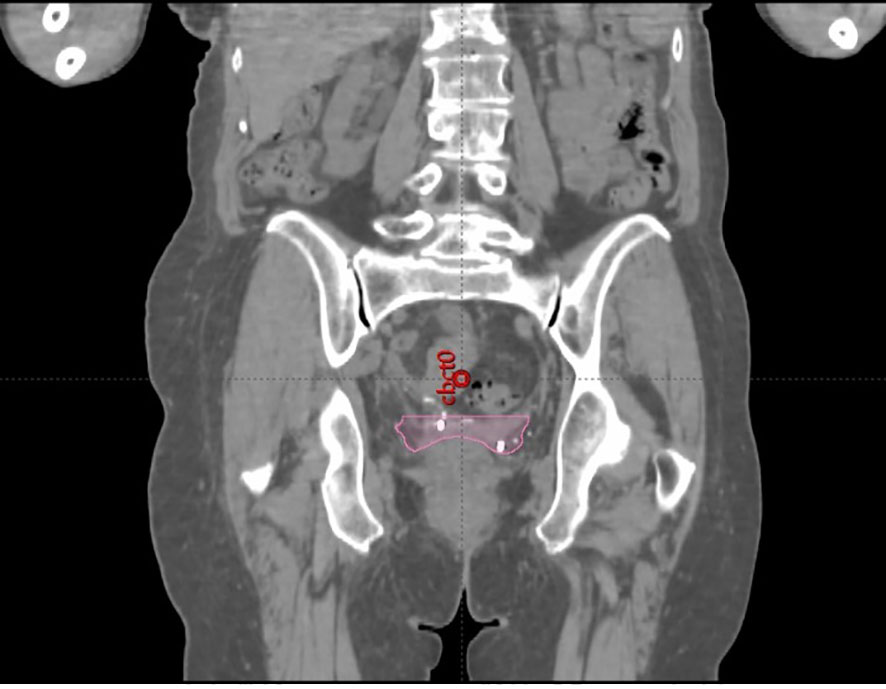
Planning CT image with the vaginal CTV with FMs.
